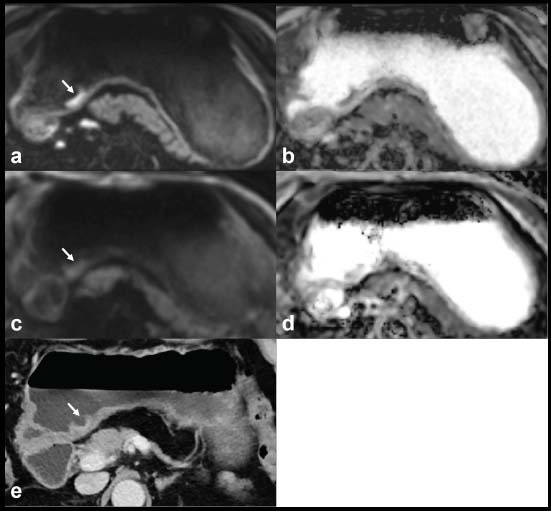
CT and MR images from a 71-year-old male patient with pathologically confirmed adenocarcinoma (stage pT1bN0M0) of the gastric antrum. The EGC tumor is clearly visible as a hyperintense region on the rFOV DWI (a, white arrow) and demonstrates hypointensity on the corresponding ADC map (b). Conversely, on the fFOV DWI, the tumor appears slightly hyperintense (c, white arrow), with hypointensity observed on the corresponding ADC map (d). CT imaging reveals focal enhancement in the inner layer of the gastric wall (e).
